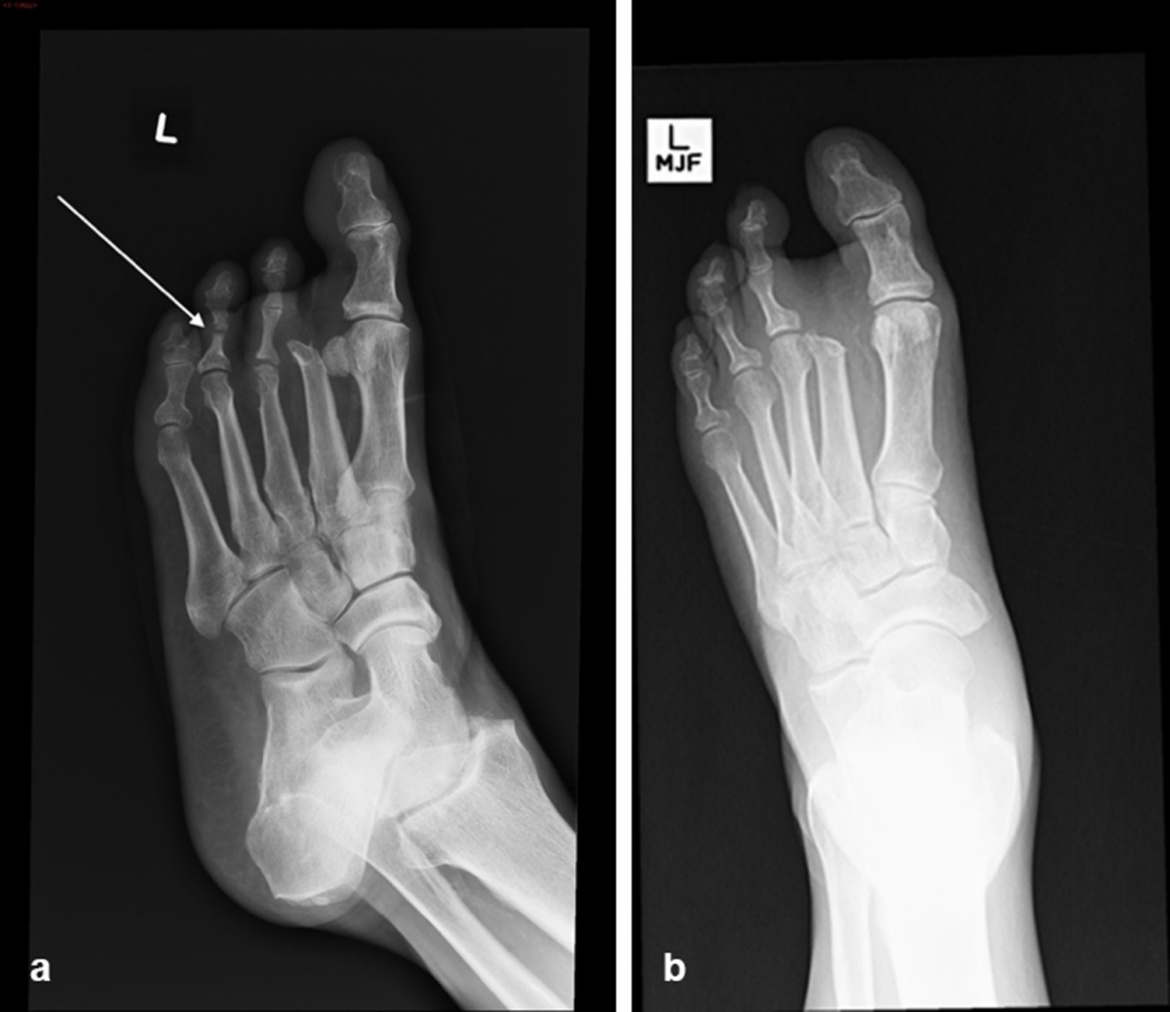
(a) Oblique radiograph of the left foot demonstrates previous amputation of the second toe and an ununited fracture of the head of the proximal phalanx of the fourth toe, probably secondary to previous trauma in an insensate foot. Furthermore, there is “pencil point” deformity of the neck of the proximal phalanx of the fourth toe (arrow). Cortical irregularity at the head of the third metatarsal raises suspicion of osteomyelitis. (b) Left foot radiograph of the same patient performed 5 years earlier, shortly after amputation of the second metatarsal, demonstrates normal appearance of the phalanges of the fourth toe.
